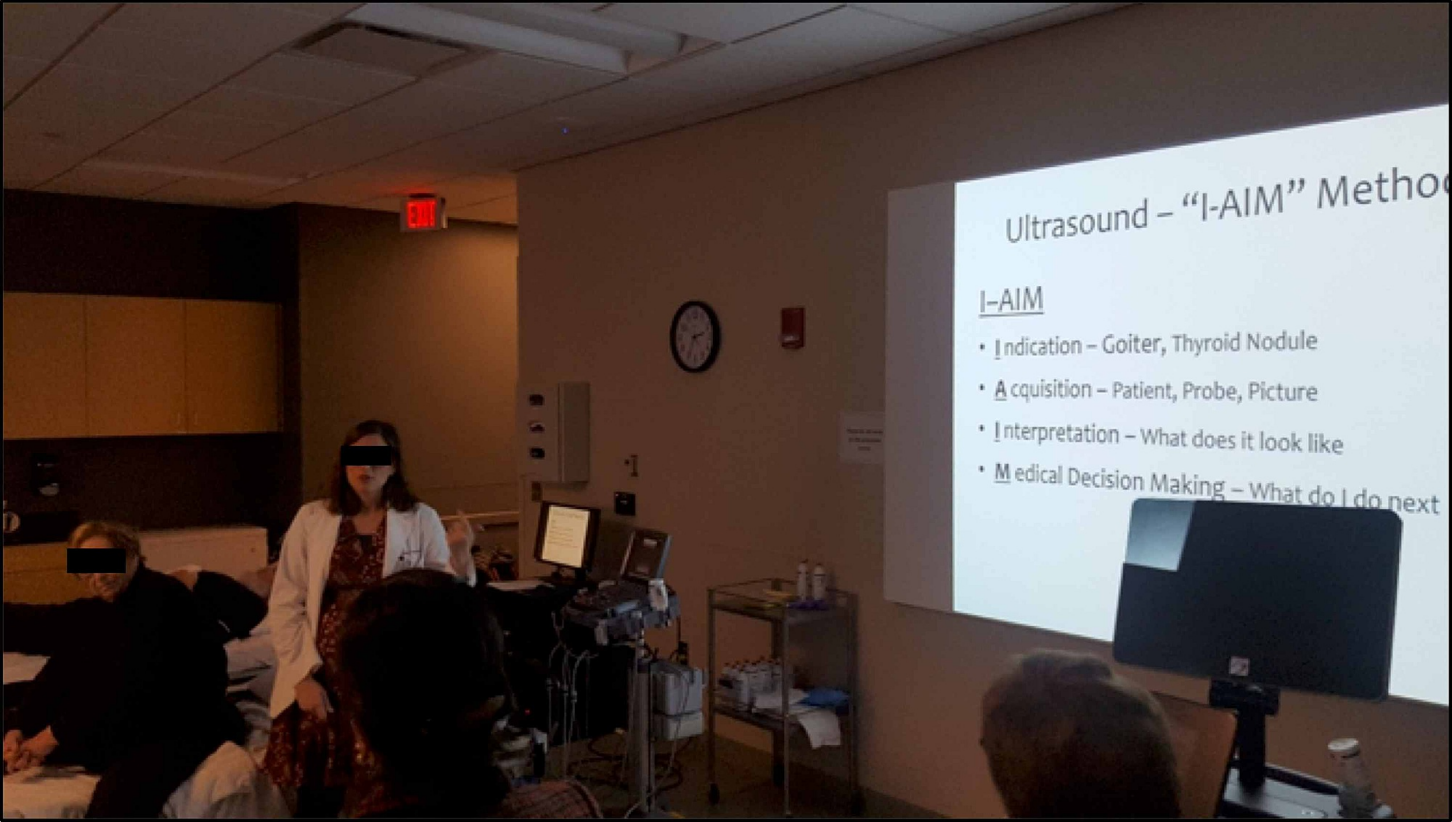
Introductory presentation covering objectives of the session, a basic introduction to ultrasound including the I-AIM technique, basic ultrasound physics, ultrasound probe types and motions, image orientation, and image description. I-IAM, indication, acquisition, interpretation, and medical decision making.
